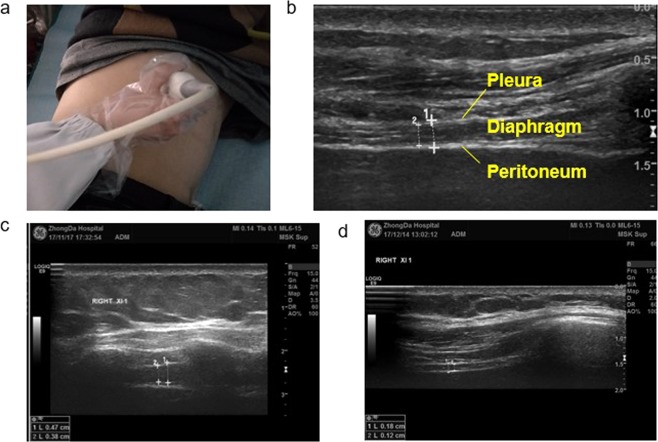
Diaphragm thickness reduction in dialysis patients during deep breathing under ultrasound. (a) The probe position for B and M mode diaphragmatic thickness measurements in the zone of apposition using a 6–13 MHz probe. (b) B-mode sonography of the diaphragm in the zone of apposition. The diaphragm is visualized as a three-layered structure comprising two parallel echogenic layers of diaphragmatic pleura and peritoneal membranes sandwiching a non-echogenic layer of the diaphragm muscle. (c) End-inspiration thickness of the diaphragm at forced vital capacity in a normal person. (d) End-inspiration thickness of the diaphragm at forced vital capacity in a dialysis patient.
